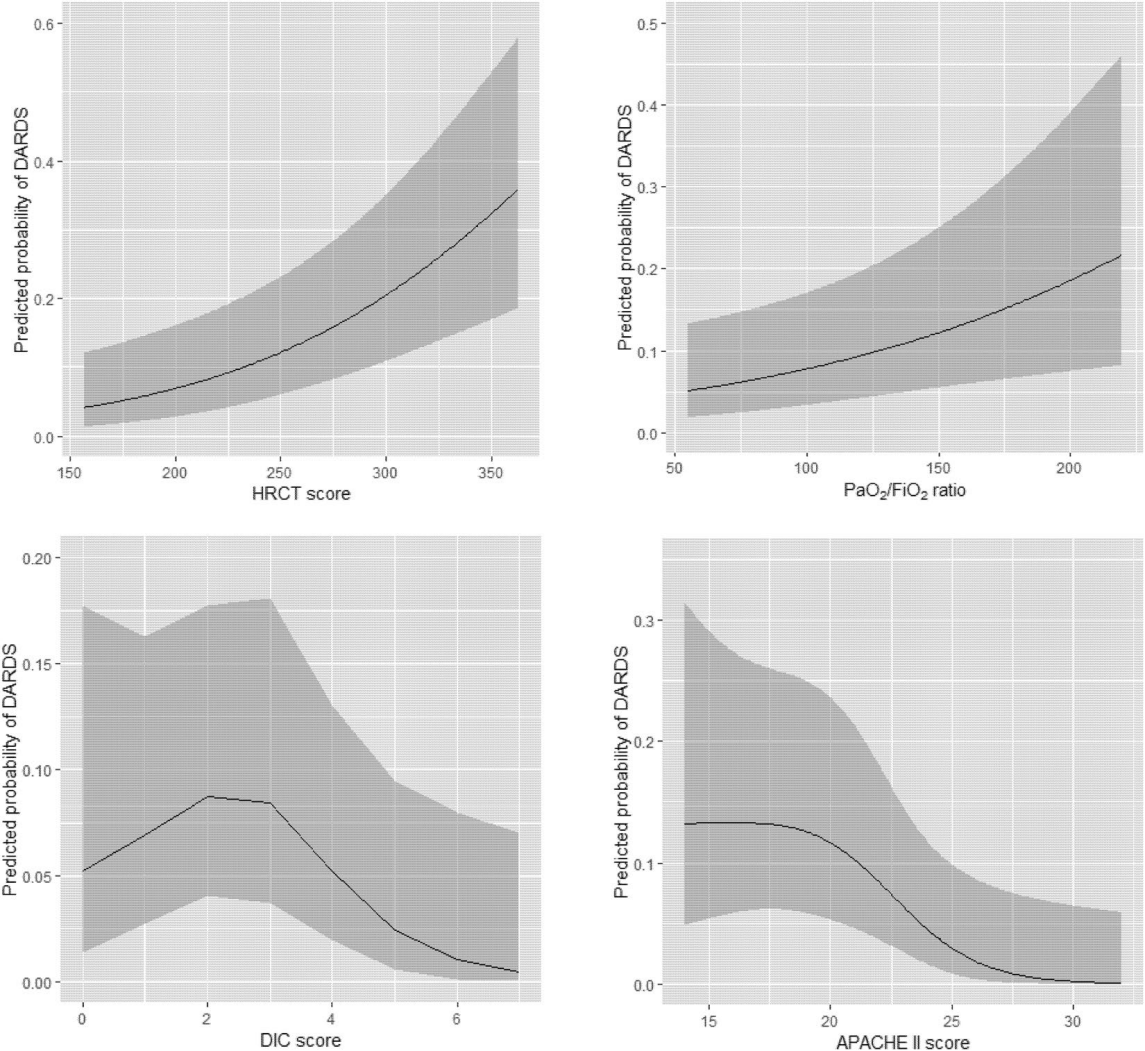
The relationship between DARDS and each variable in the HRCT added model.
